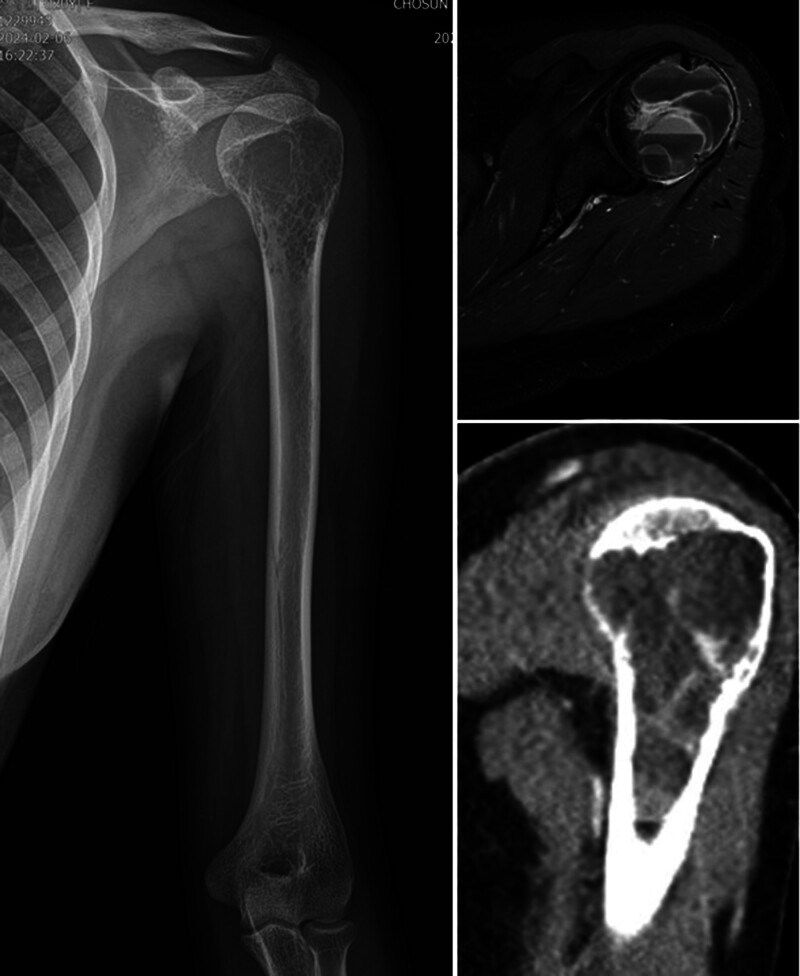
Preoperative images of the left shoulder. A plain radiograph (left) shows an osteolytic intramedullary lesion in the left proximal humeral epi-metaphysis. T2 axial magnetic resonance image shows multiple fluid-fluid levels in the lesion (right upper). The coronal view of computed tomography (CT) image (right lower) shows poorly demarcated multilocular osteolytic intramedullary mass with preserved humeral cortex.
